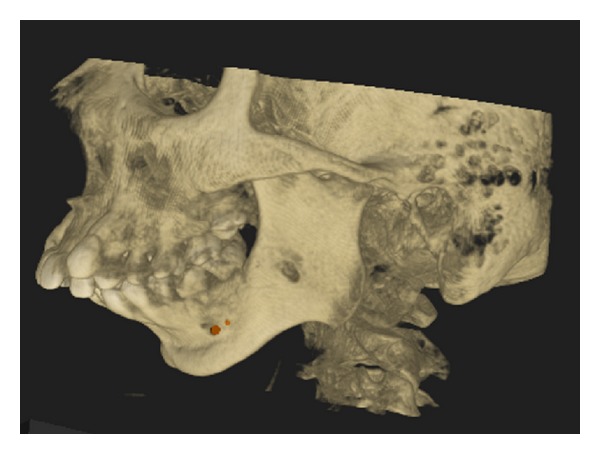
3D CBCT left lateral—shows hypoplasia of glenoid fossa and condyle.
